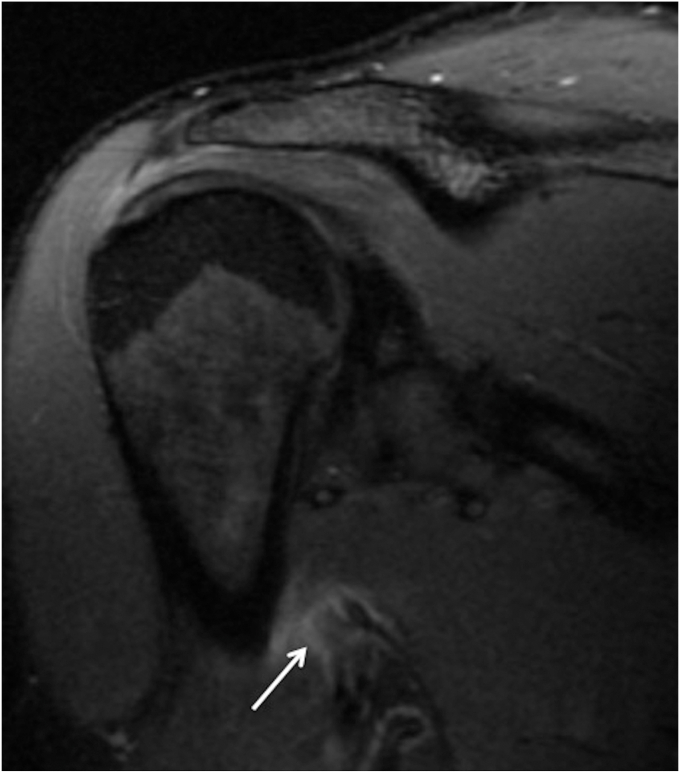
Coronal MRI of grade IIIA latissimus dorsi/teres major tear with retraction of tear and surrounding edema (*Arrow*). *MRI*, magnetic resonance imaging.
